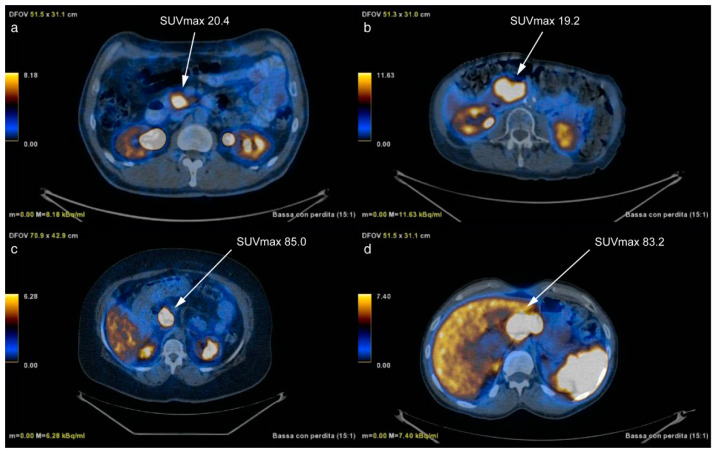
[68Ga]Ga-DOTANOC PET/CT transaxial fused images of two pairs of patients presenting similar SUVmax values of the primary lesion but different tumour grades. In particular, although SUVmax of (a,b) is similar, (a) is G1 while (b) is G2; accordingly, (c,d) present similar SUVmax primary tumor values but (c) is G1 while (d) is G2. On the contrary, the primary lesion’s grade was correctly identified (G1: a,c; G2: b,d) when using the selected RFs.
